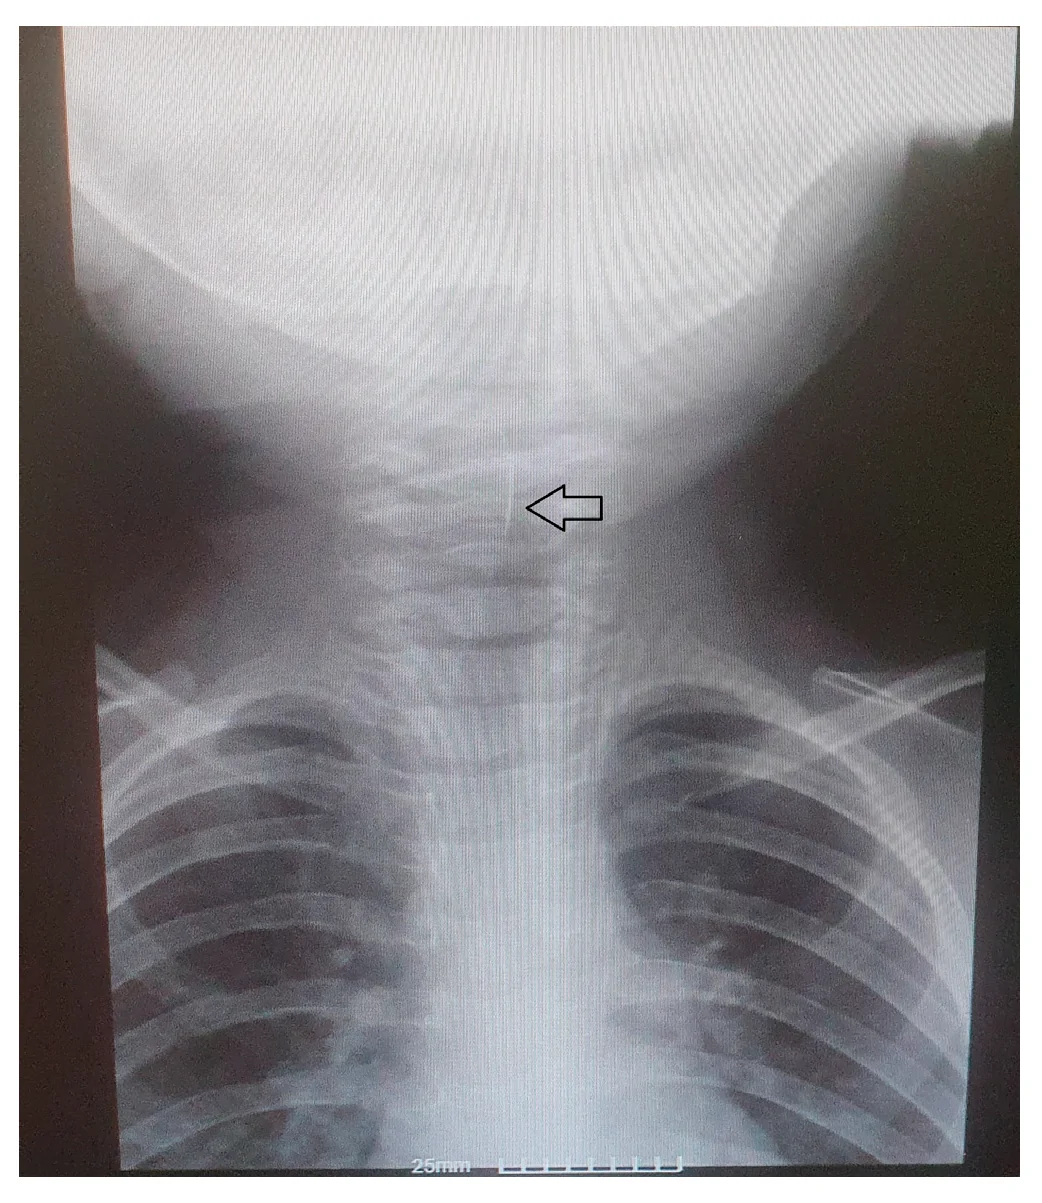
Anteroposterior neck radiograph showing a radiopaque foreign body (arrow) at the level of the larynx, later confirmed endoscopically to be an eggshell fragment.
